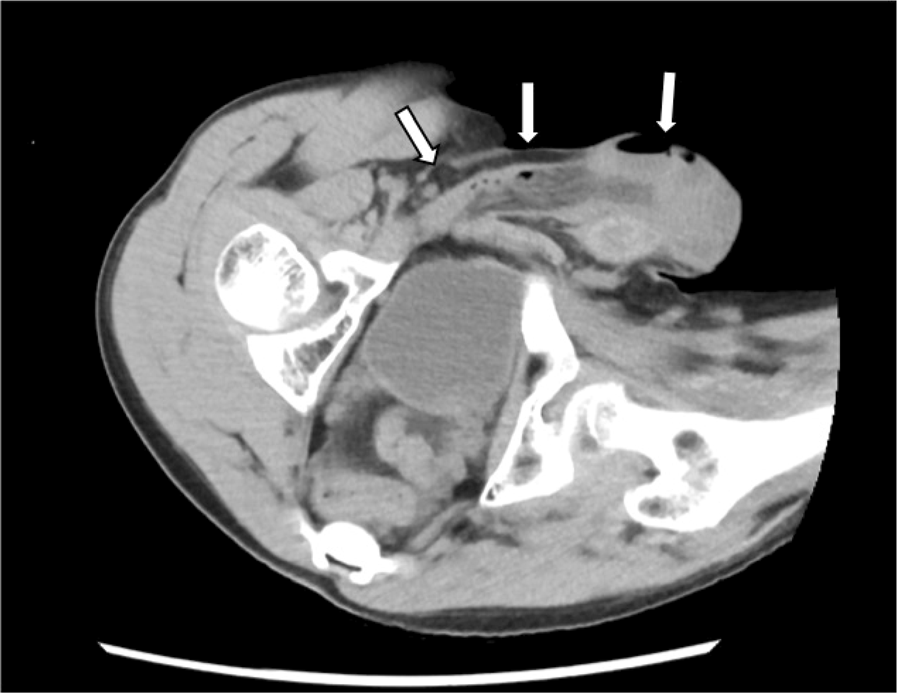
Computed tomography showed an indirect inguinal hernia on the right side
